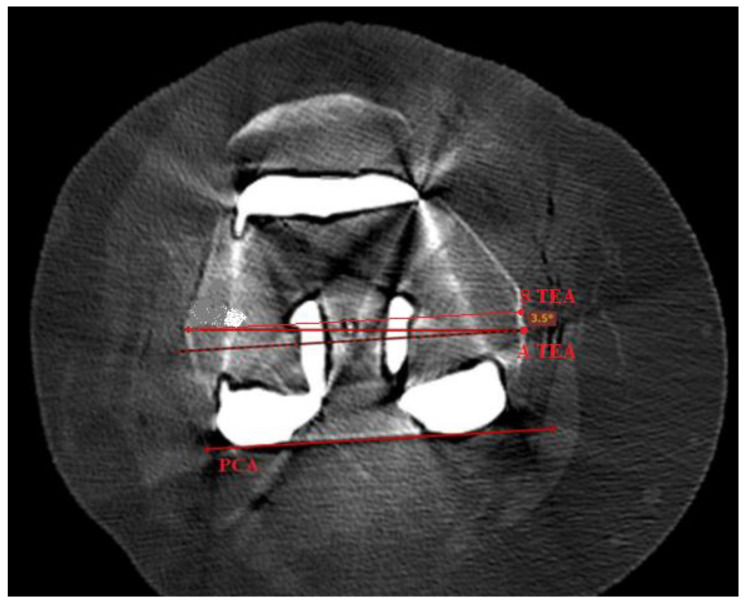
Postoperative TKA CT scan of one of the patients analyzed in the study. It reveals the graphical representation of S TEA and PCA lines, utilized and described in the study methods.
